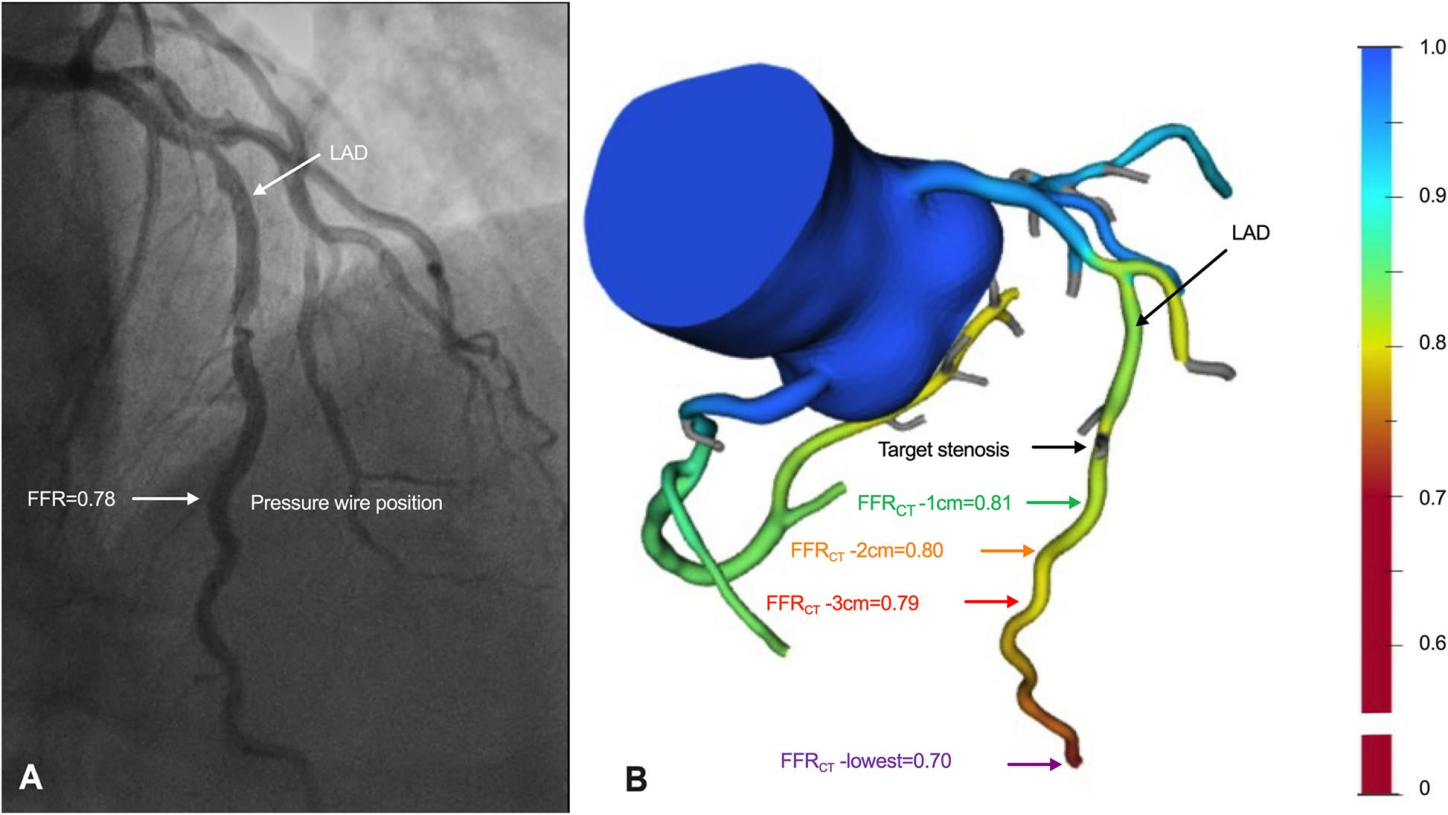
Representative images for invasive FFR and FFR_CT_ measurements. A ICA image showing the tip of pressure-wire position in left anterior descending artery (LAD) during invasive FFR and the measured FFR was 0.78. B The corresponding pseudo-colorized FFR_CT_ image show the site of target lesion and the measured FFR_CT_ at 1, 2, 3 cm distal to the target lesion (FFR_CT_-1 cm, FFR_CT_-2 cm FFR_CT_-3 cm) and the lowest FFR_CT_ (FFR_CT_-lowest). LAD, left anterior descending artery; FFR, fractional flow reserve; FFR_CT_, CT fractional flow reserve; FFR_CT_-1 cm, FFR_CT_ measured at 1 cm distal to the lower border of the target lesion; FFR_CT_-2 cm, FFR_CT_ measured at 2 cm distal to the lower border of the target lesion; FFR_CT_-3 cm, FFR_CT_ measured at 3 cm distal to the lower border of the target lesion; FFR_CT_-lowest, lowest FFR_CT_ value in the distal vessel tip
